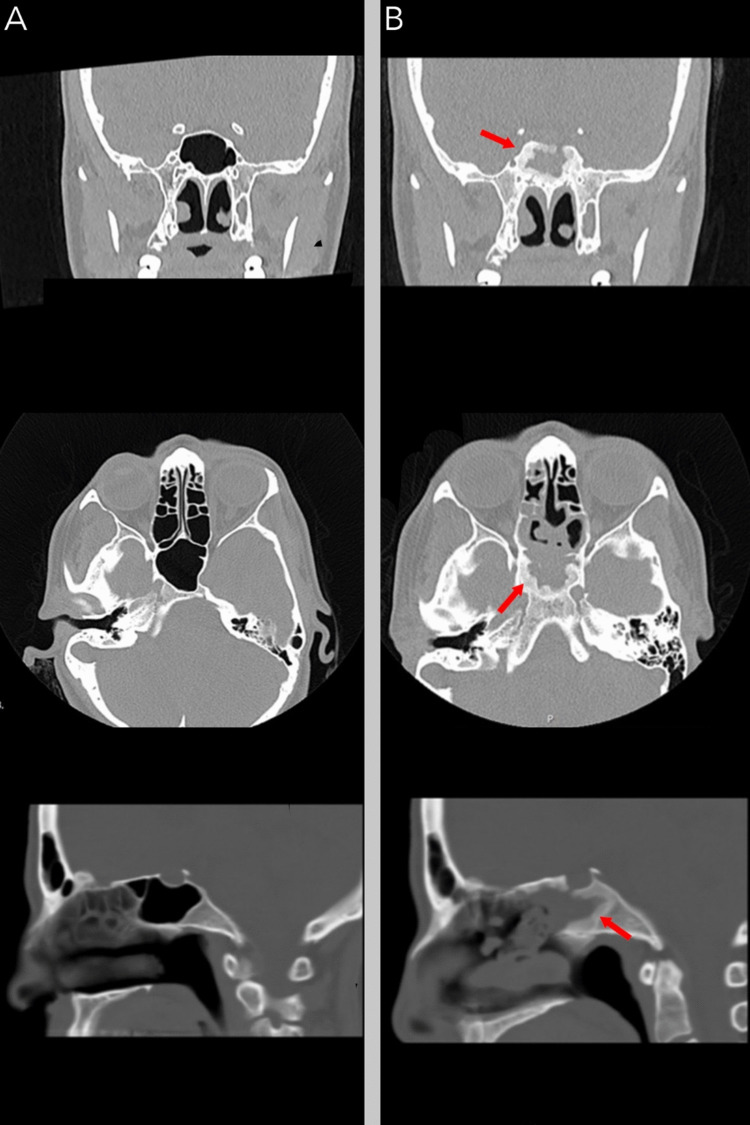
Preoperative (A) and postoperative (B) computed tomography (CT) images in axial, coronal, and sagittal planes of Patient 12, showing marked bony thickening and sclerosis consistent with Lee–Kennedy Grade 3 sphenoid sinus osteitis. Red arrow: Bony thickening
